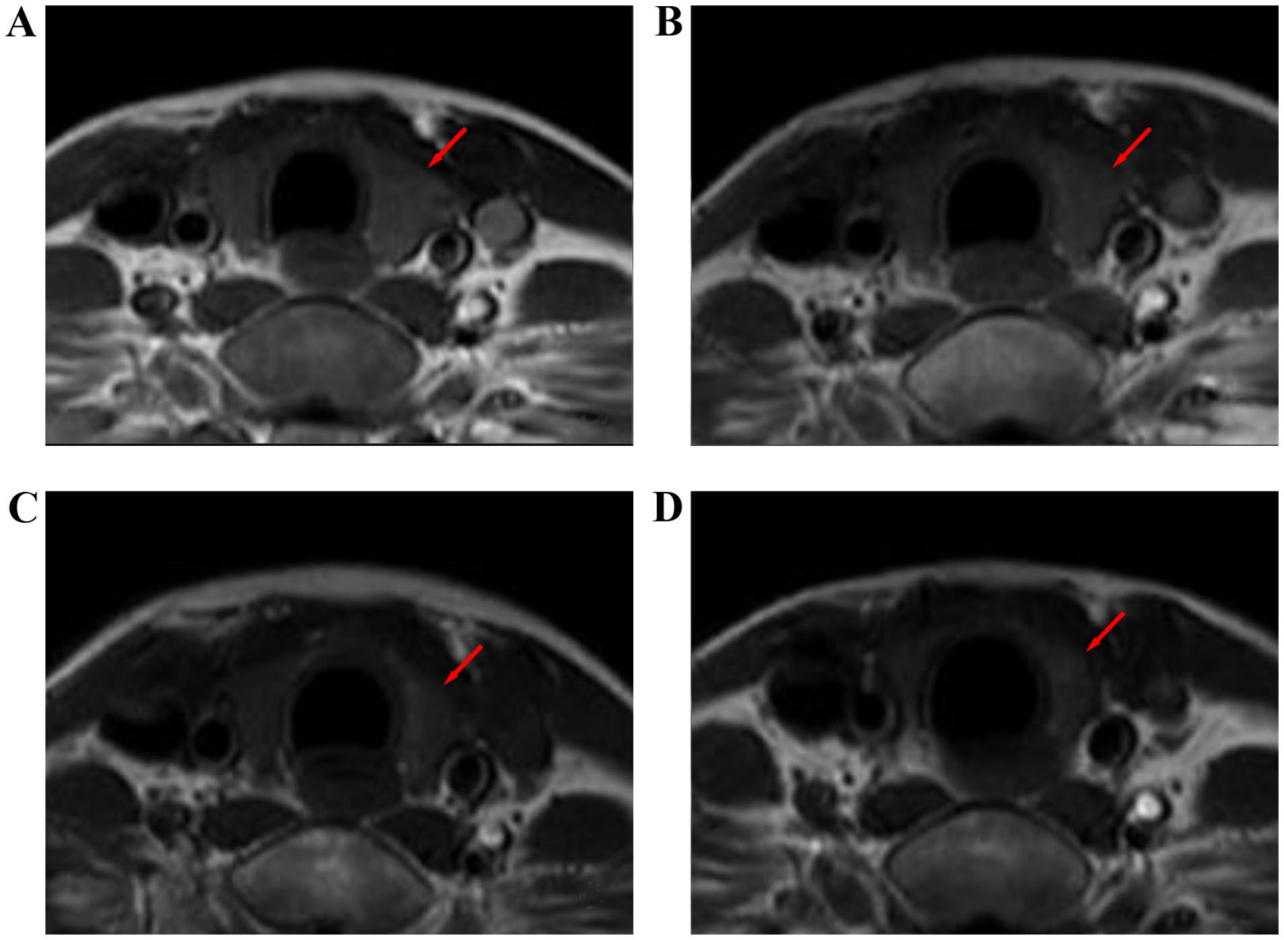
T1-weighted images of the thyroid gland of a 32-year-old female with nasopharyngeal carcinoma before and after radiotherapy. (A) Before radiotherapy, (B) during radiotherapy, (C) 6 months after radiotherapy, and (D) 12 months after radiotherapy.
